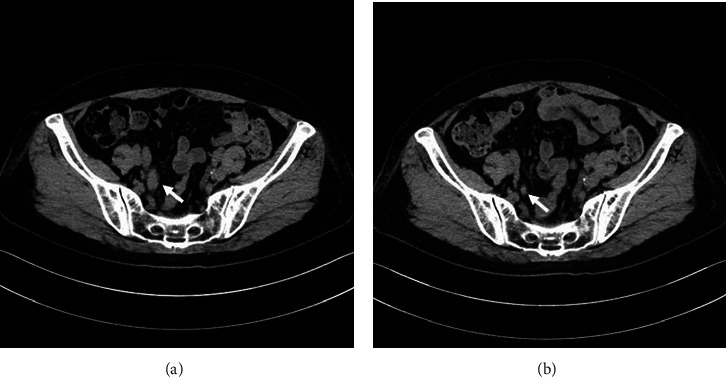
CT findings. (a) Plain CT at diagnosis shows a right invasive ureteral tumor and no metastatic findings ((a) arrow). (b) Plain CT after four course of chemotherapy shows the right ureteral tumor diminished in size after the therapy ((b) arrow).
